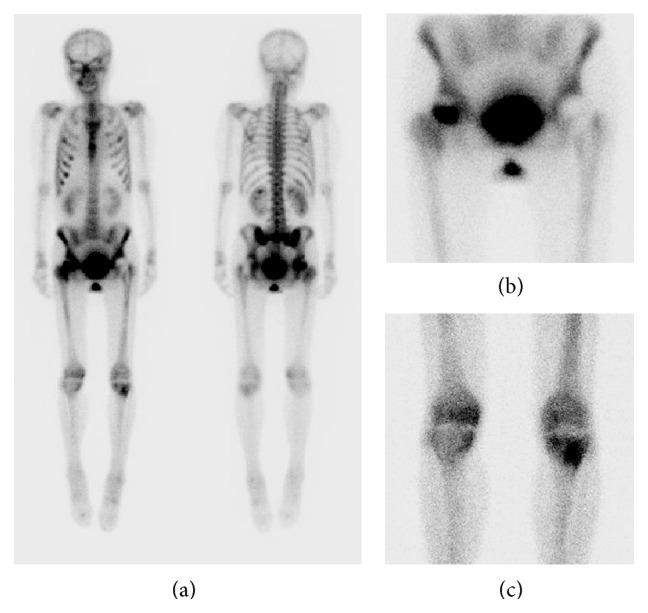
Bone scintigram showed the abnormal uptake in the right hip (a, b) and the right and the left knee joints (a, c).
